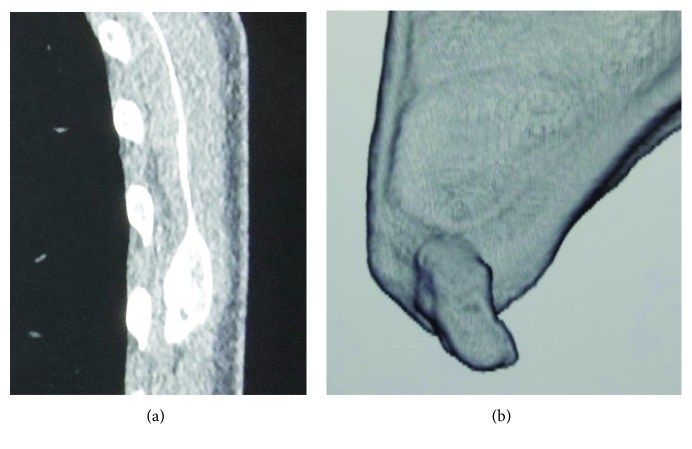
Computed tomography at the time of the second visit 4 months later. (a) Sagittal section revealing the disappearance of the narrow space between the fragment and the scapular body. (b) Three-dimensional reconstruction image showing the disappearance of the narrow break between the protrusion and the fragment.
